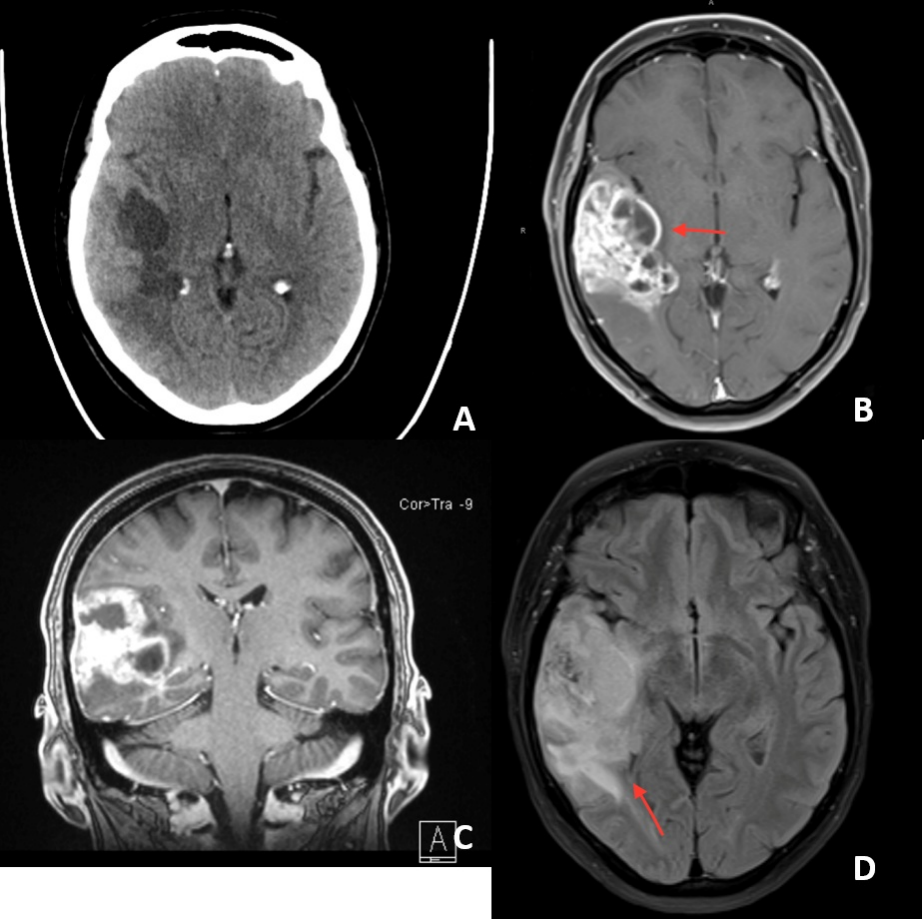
Imaging at emergency room admission A) Axial computed tomography (CT) scan showing hypodensity in the right hemisphere suggesting right middle cerebral artery stroke; B) Axial T1 magnetic resonance imaging (MRI) sequence with contrast showing mixed cystic and solid (arrow) enhancing right-sided temporal lesion concerning for brain neoplasm. The lesion involves the superior temporal gyrus anteriorly and all three temporal gyri posteriorly with minimal infiltration into the inferior most gray matter at the opercular border; C) Coronal section of the same sequence; D) Fluid-attenuated inversion recovery (FLAIR) sequence and the associated edema (arrow).
